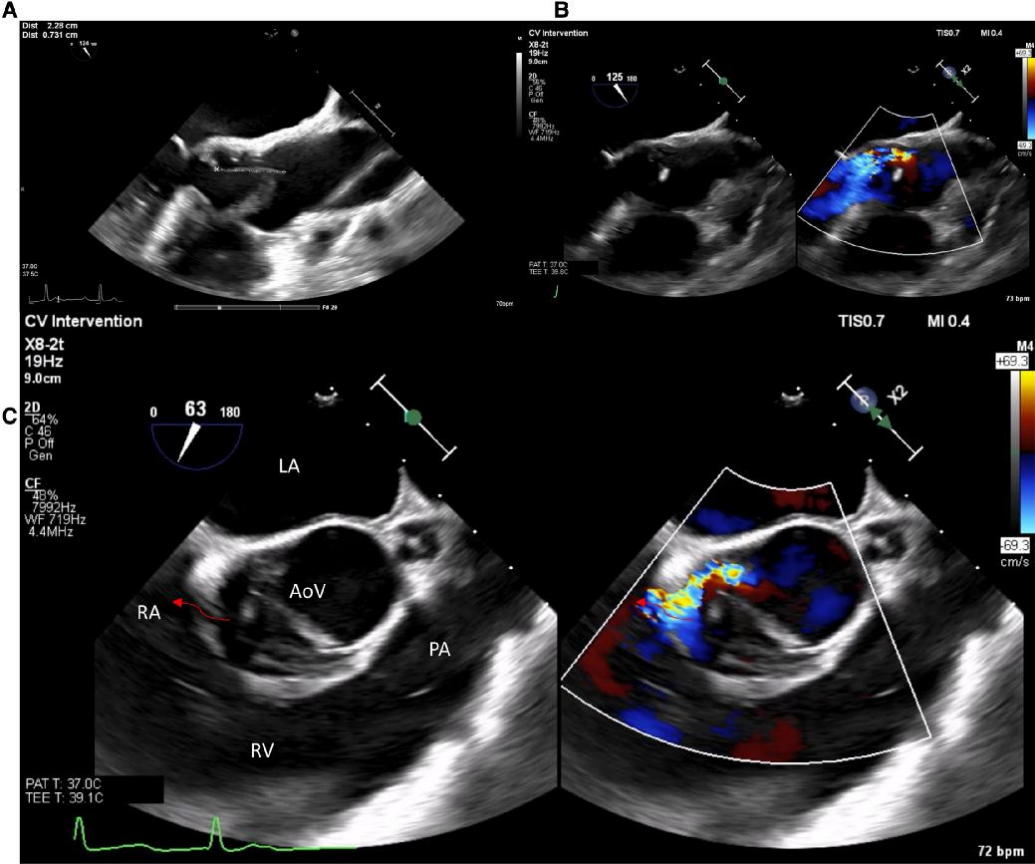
TOE: (*A*) filiform highly mobile mass attached to the mitral–aortic intervalvular fibrosa with dimensions of 22.8 × 7.3 mm, (*B*) moderate aortic regurgitation due to perforation of the posterior/left cusp, and (*C*) LVOT-RA communication (arrow) with high velocity jet at Doppler evaluation (Gerbode defect).
